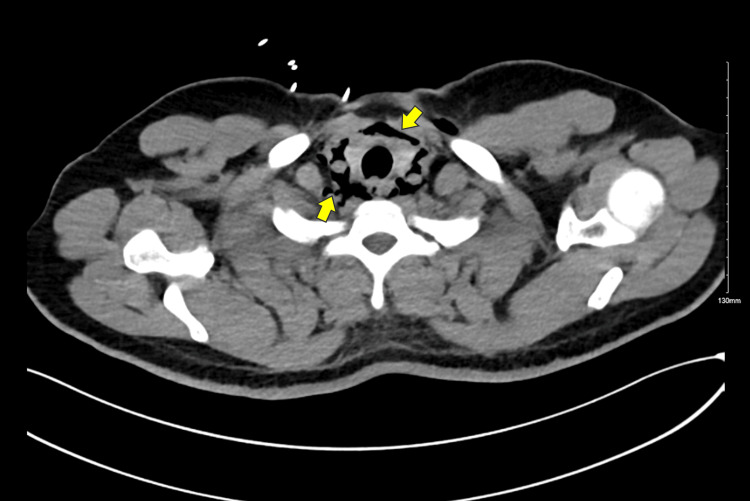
Axial chest CT with soft tissue window showing pneumomediastinum (arrows) CT: computed tomography
